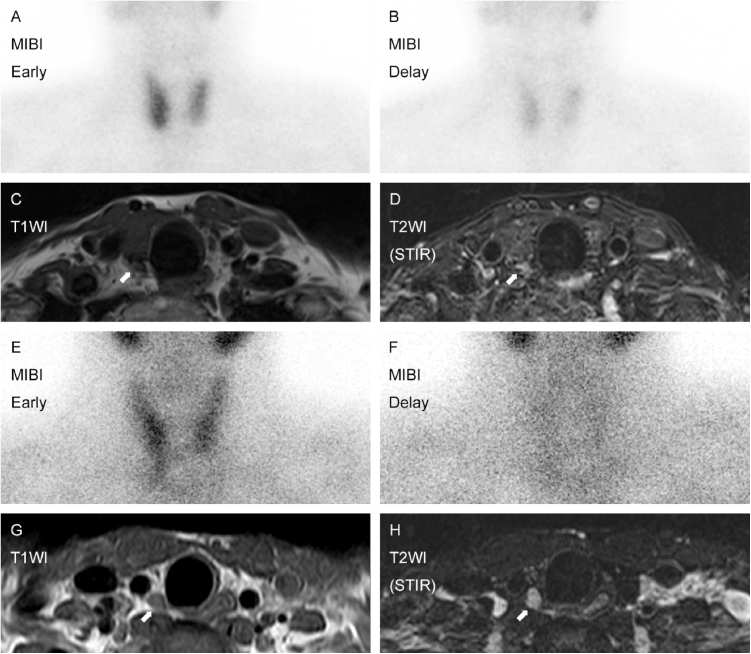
Two cases detected by MRI which were not initially seen on US. Initial case was a 76-year-old female with 8 mm adenoma. MIBI presented diffuse uptake on the right side (A and B). Not US but MRI revealed the lesion (C and D). Another case was a 35-year-old male with 17 mm adenoma. MIBI represented equivocal accumulation on the right inferior quadrant (E and F). The lesion was successfully detected by MRI (G and H), which were not initially localized on US.
